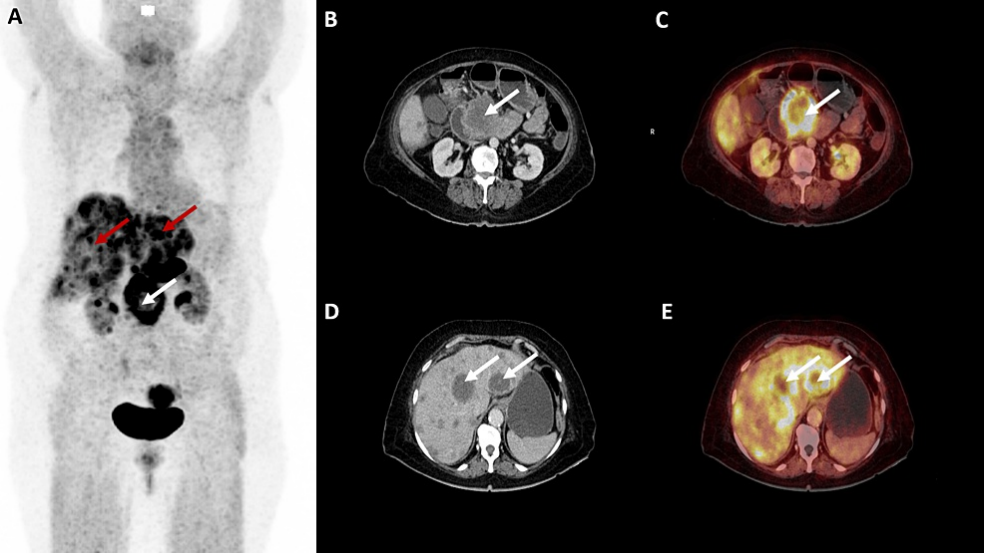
68Ga-FAPI PET/CT images of the patient (A) MIP; (B, D) axial view of CT; (C, E) axial view of 68Ga-FAPI PET/CT fusion. MIP image shows increased FAPI uptake in the region of the pancreas (white arrow) and metastatic liver lesions (red arrows). (B, C) Primary neoplastic lesion involving head and uncinate process of pancreas with FAPI uptake (white arrows). (D, E) Metastatic liver lesions with FAPI uptake (white arrows). MIP: maximal intensity projection; 68Ga-FAPI PET/CT: 68Ga-fibroblast activation protein inhibitor positron emission tomography/computed tomography; FAPI: fibroblast activation protein inhibitor.
